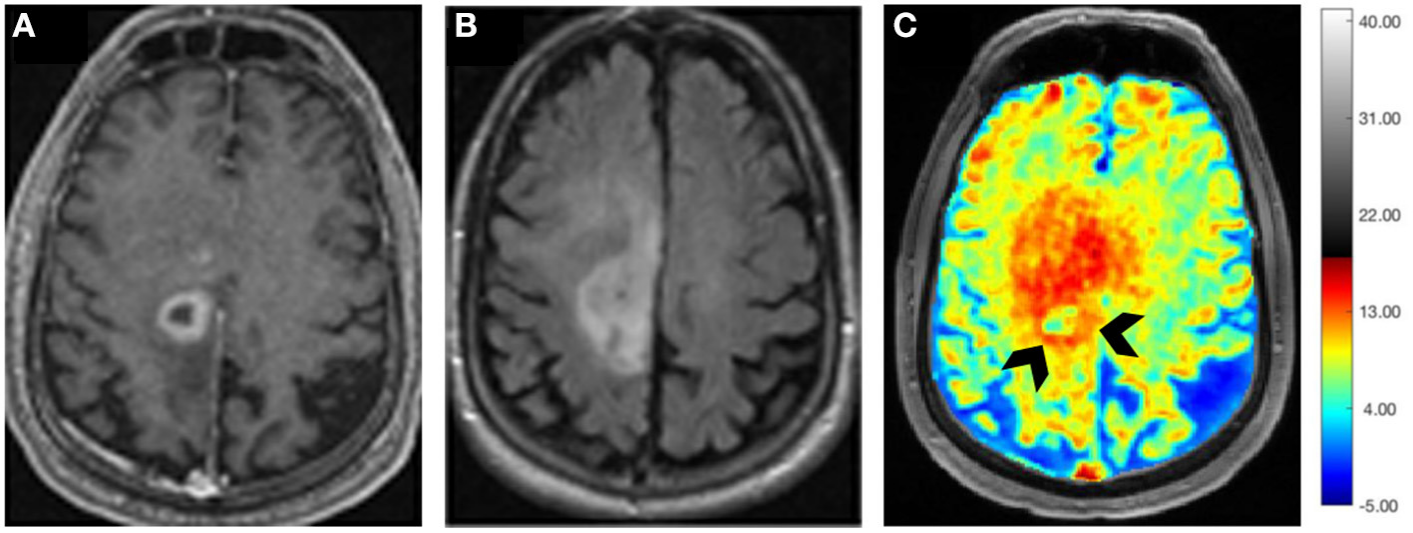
Axial postcontrast T_1_ weighted image (A) demonstrating a ring-enhancing GBM with hypointense vasogenic edema in the left frontal lobe, which is visible as a hyperintense mass on the corresponding T_2_-FLAIR image (B). B_0_ and B_1_ field in-homogeneity corrected Glu-CEST map (C) showing high glutamate signals from contrast-enhancing ring (arrowheads) and the surrounding brain regions beyond the contrast enhancement, suggesting infiltrative nature of this neoplasm.
